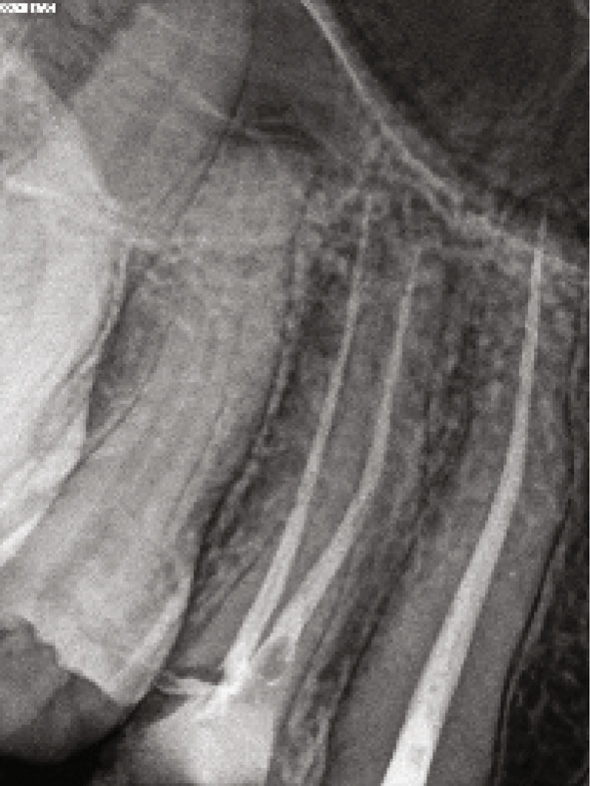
Periapical radiograph following the completion of root canal treatment.
